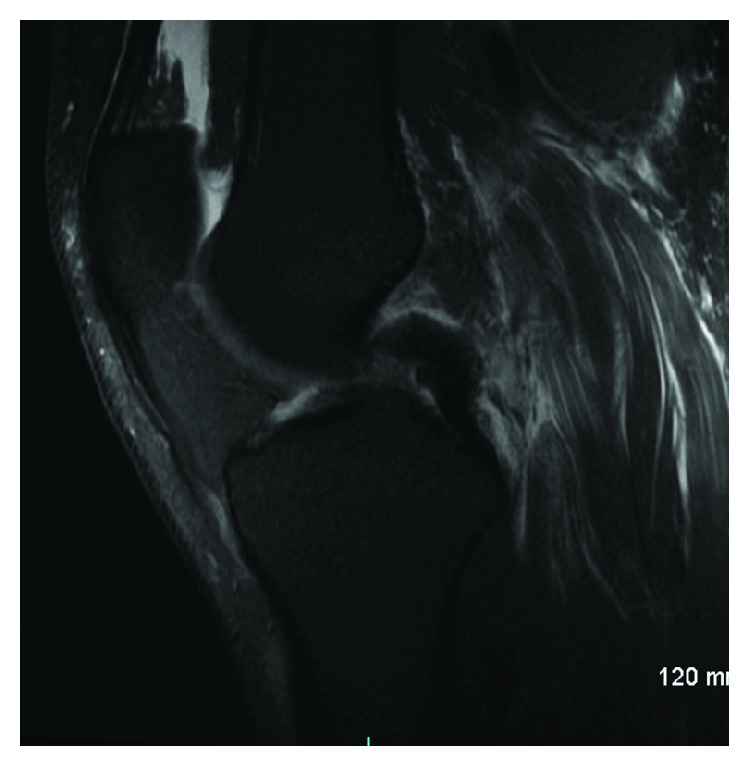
A T2 sagittal MRI of the patient's right knee demonstrating a grade II PCL tear.
